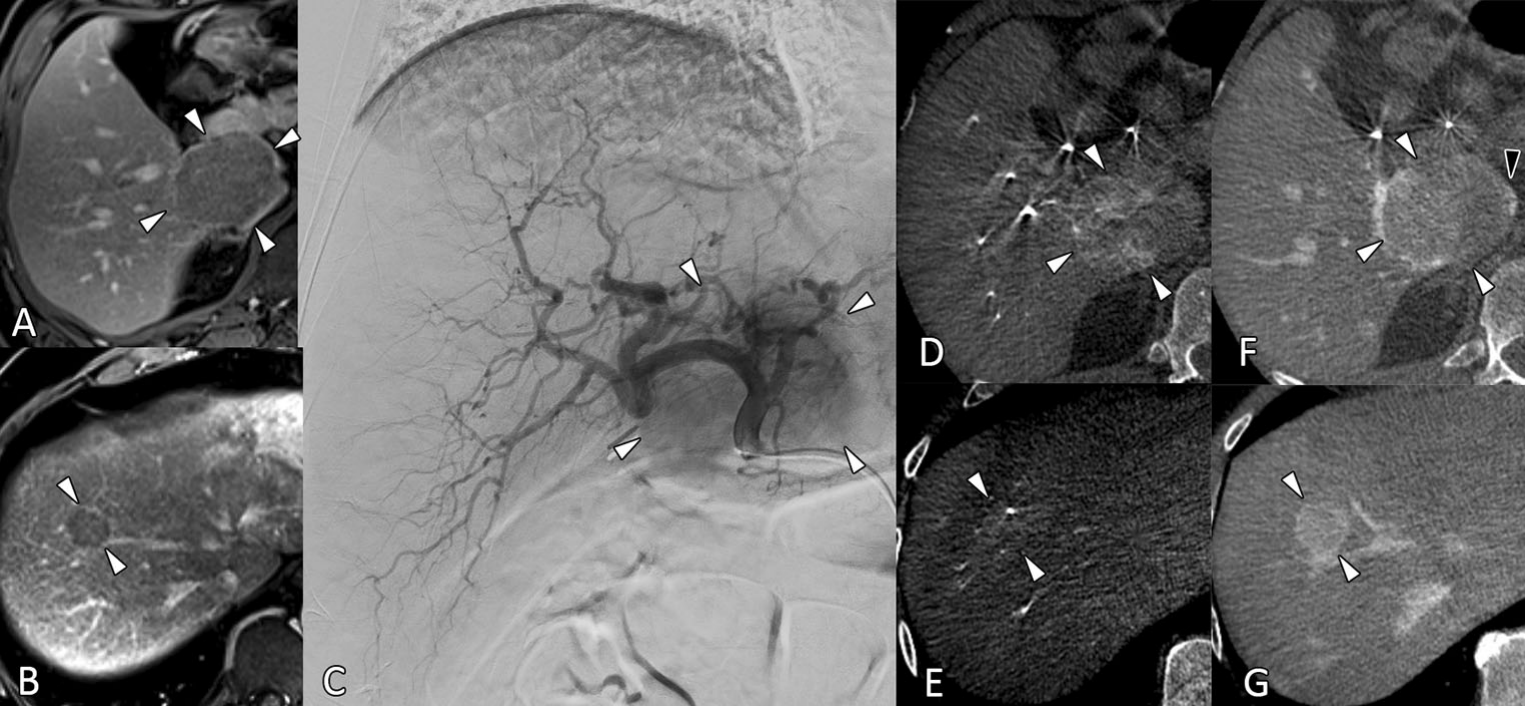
54-year-old man with a history of neuroendocrine cancer of the small bowel with liver metastases, treated using conventional TACE. Contrast-enhanced T1-weighted gradient-echo sequence in the portal venous phase shows a large, rim-enhancing lesion in the caudate lobe (A, *arrowheads*) and a smaller lesion of similar pattern in segment 8 (B, *arrowheads*). On DSA images acquired with the microcatheter tip in the proper hepatic artery, only the large lesion could be identified (C, *arrowheads*). On early arterial phase CBCT images, only the lateral parts of the large lesion are depicted (D, *arrowheads*), the smaller lesion is only silhouetted against the surrounding parenchyma (E, *arrowheads*). On delayed arterial phase CBCT images, the large lesion is well depicted (F) to include both the lateral parts (*white arrowheads*) as well as the medial rim (*black arrowhead*). Of note, the small lesion is completely depicted (G, *arrowheads*)
